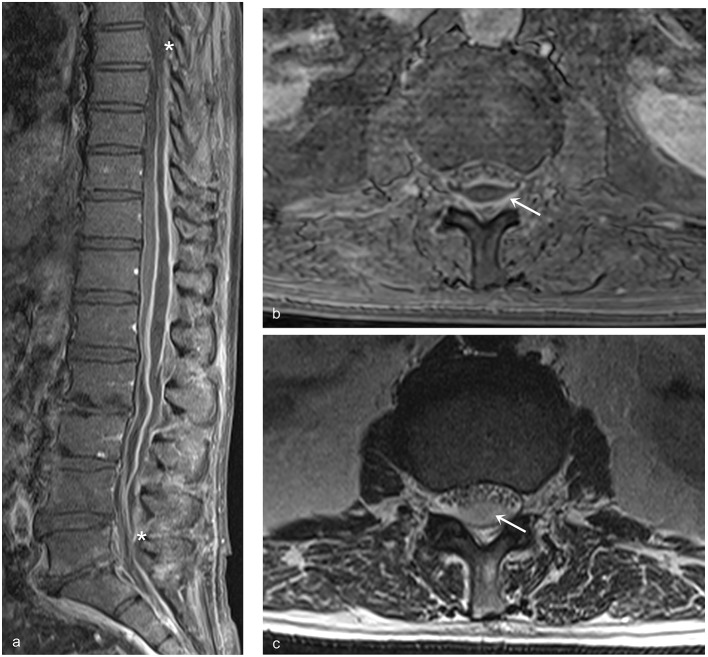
Sagittal and axial MRI images of patient no. 3. (a) The extensive posterior SEA extending from Th7 to L5 is delimited by an enhancing pseudo-capsule. The asterisks indicate the cranial and caudal limits of the lesion compressing anteriorly both the spinal cord and the cauda equina. (b) Axial MRI image T1 weighted with contrast. The arrows indicate the enhancing capsule of the SEA; psoas and paravertebral muscles appear normal. (c) Axial MRI image T2 weighted. The arrow indicates the purulent content of the SEA; no edema is visible in psoas and paravertebral muscles.
